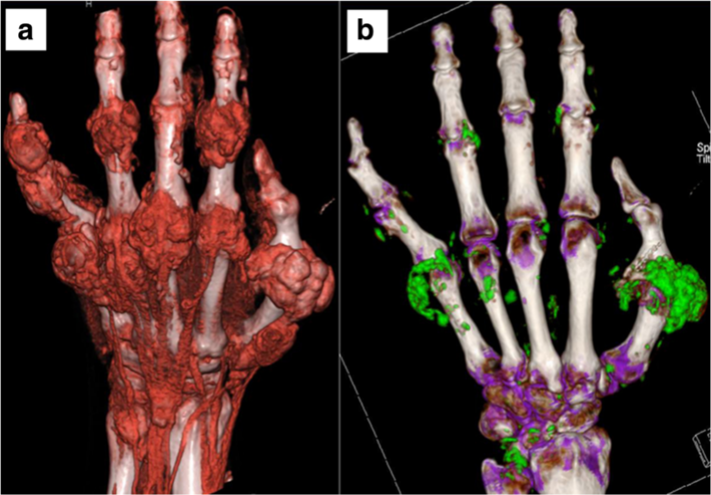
Patient 20. Large tophi are evident in the fingers with 3D volume rendering of the 2-D CT images, using proprietary software for the digital reconstruction and application of colors and varying degrees of transparency to the tissues panel (a). With DECT scanning panel (b), MSU deposits (evident by their green coding) conform to the areas of tophaceous deposits, but are sparse in some affected areas and absent in others (e.g. little finger DIP and long finger PIP)
